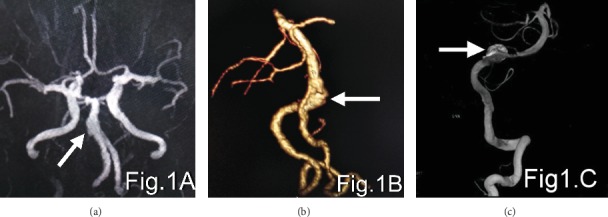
(a–c) show the MRA, CTA, and 3D-DSA, respectively, of basilar artery aneurysms in the same patient. The arrows show the aneurysm.
